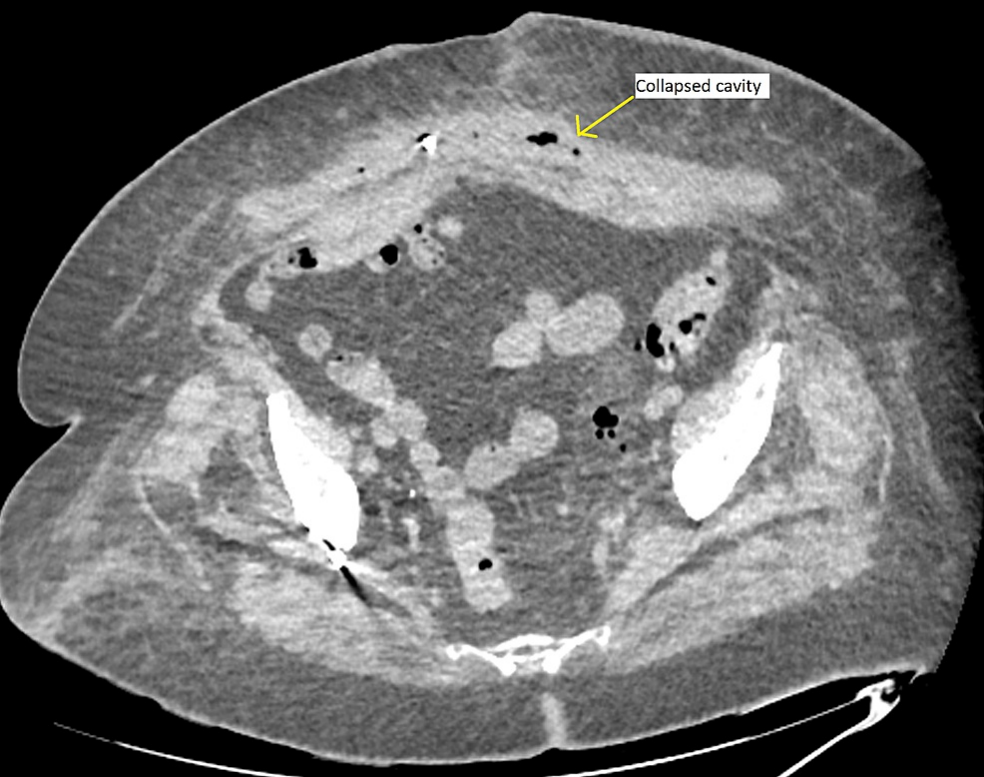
CT abdomen post drainage (axial slice).
